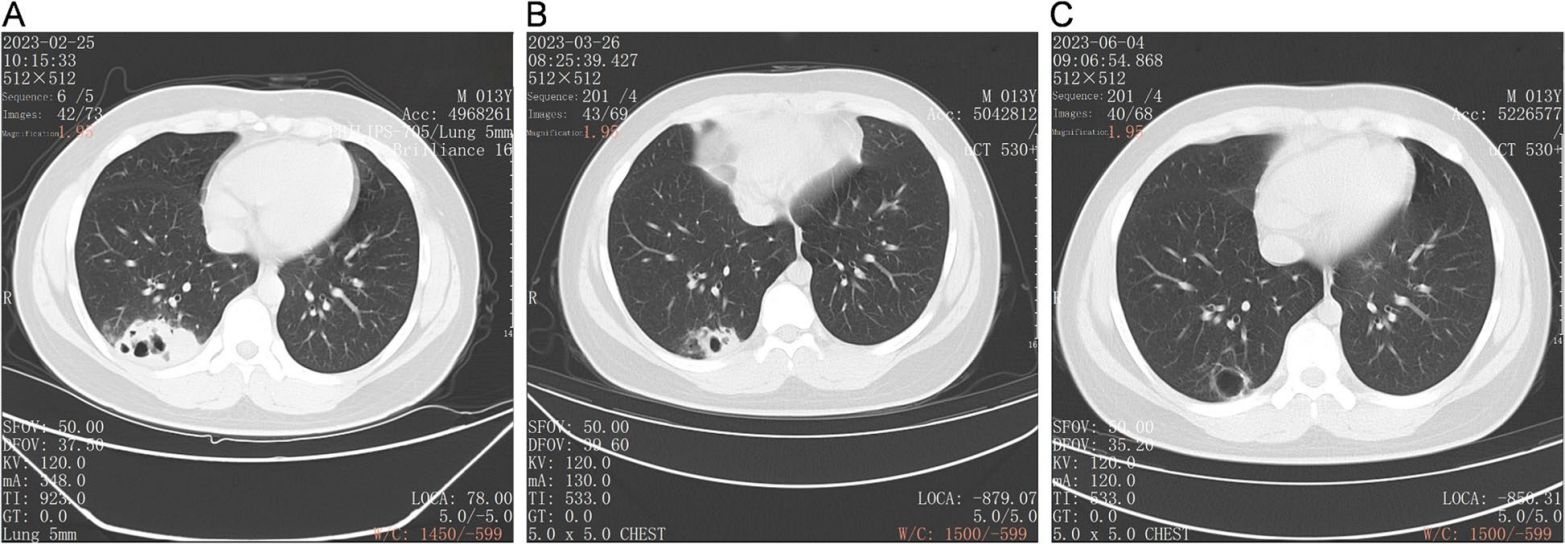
Images. a CT on admission showed a lesion and cavities in the right lower lobe of the lung, suggesting infection. b CT on the recovery phase (after taking fluconazole orally for 1 month) showed the lesion in the lower lobe of the right lung had reduced. c CT on the recovery phase (after taking fluconazole orally for 4 month) showed the inflammation in the lower lobe of the right lung had well absorbed
